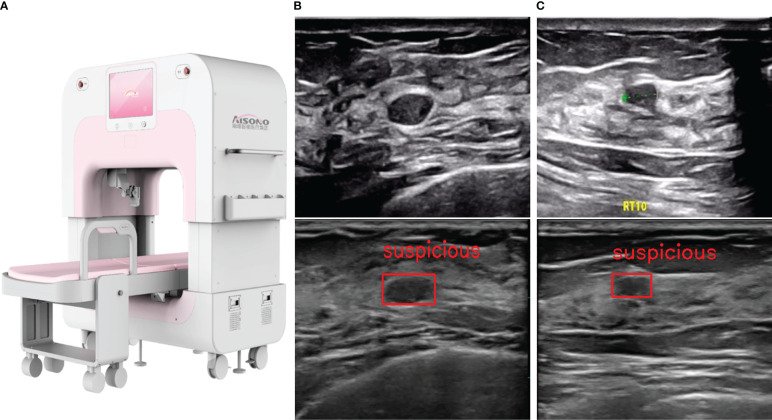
AIBUS ultrasound machine and images. (A) The machine appearance of AIBUS (B) HHUS (top) and AIBUS (bottom) image of example 1: a 46-year-old woman with breast mass that was interpreted as BI-RADS 3 (C) HHUS (top) and AIBUS (bottom) image of example 2: a 57-year-old woman with breast mass that was interpreted as BI-RADS 3.
